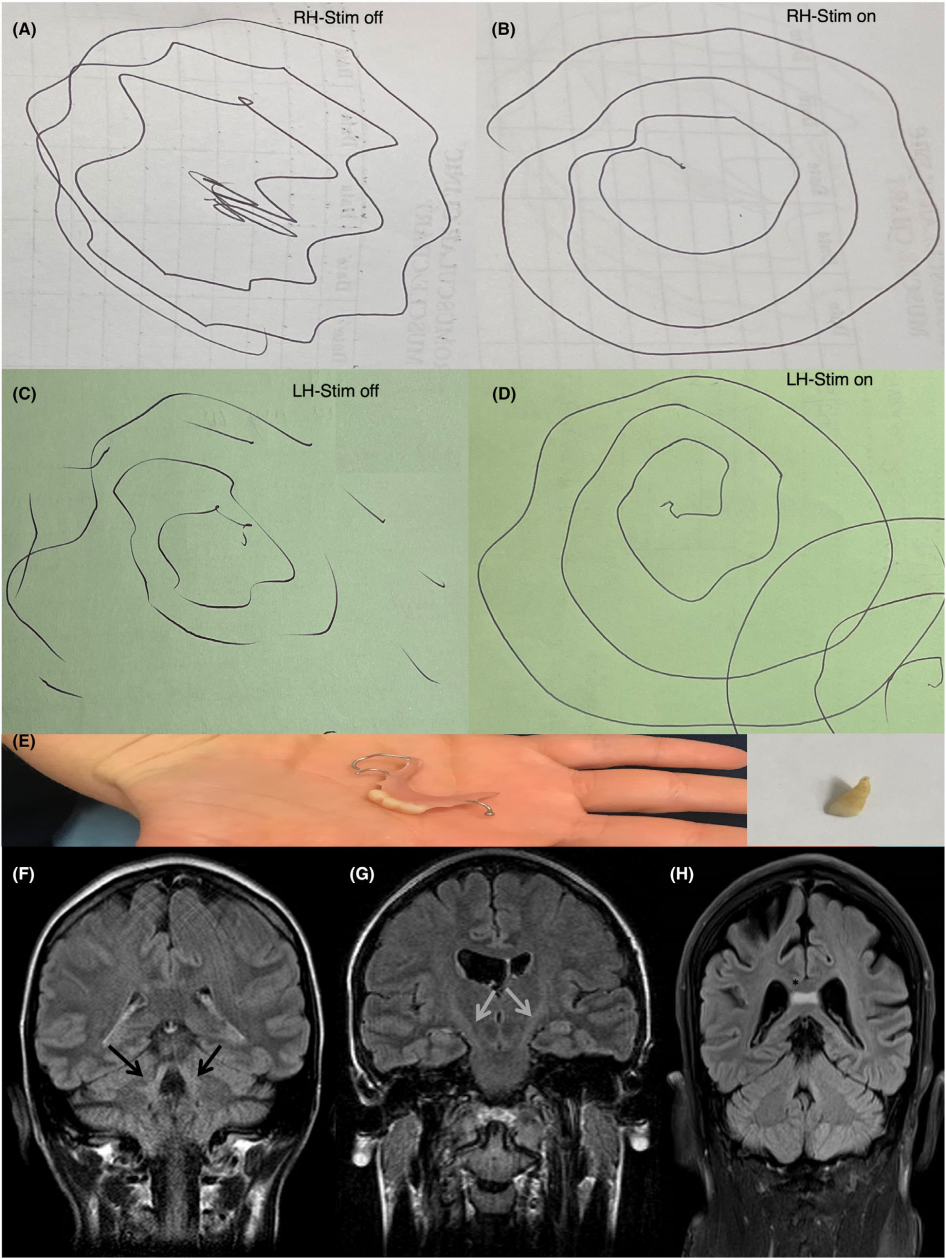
Archimedean spiral of patient D1 with DBS on/off (A–D); denture of patient D2 with insert showing her one of her small teeth (E); MRI head coronal FLAIR of patient F1 showing SCP sign (F), patient D1 showing pyramidal tract hyperintensities (G), and patient C1 showing splenium of corpus callosum hyperintensities (H).
